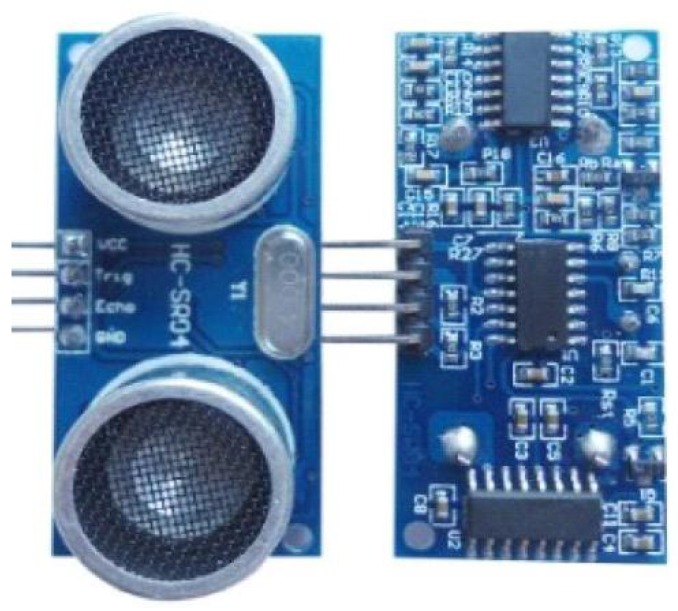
Ultrasound module of HC-SR04: Front (left) and back (right) faces.
